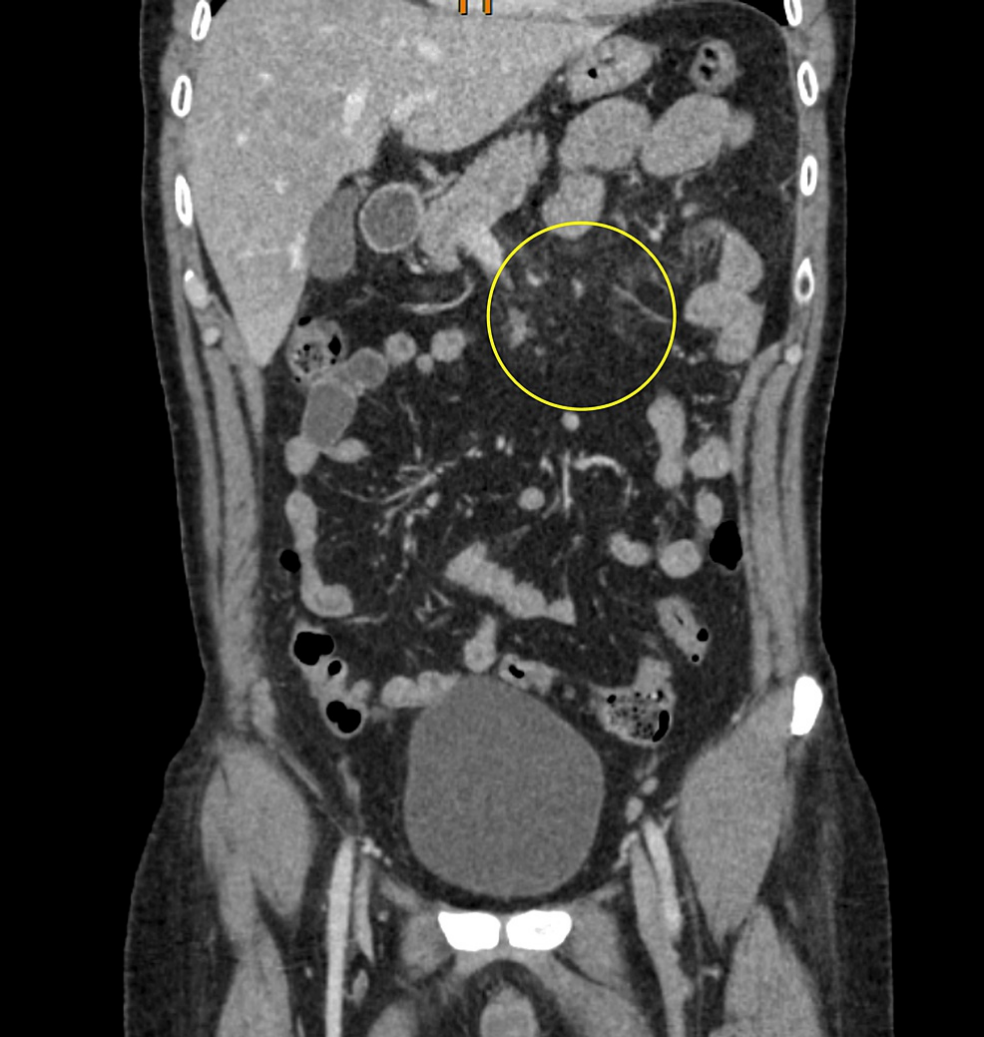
Coronal computed tomography of the abdomen with intravenous contrast The yellow circle shows mild haziness of the small bowel mesentery (misty mesentery) seen at the left hypochondrium.
